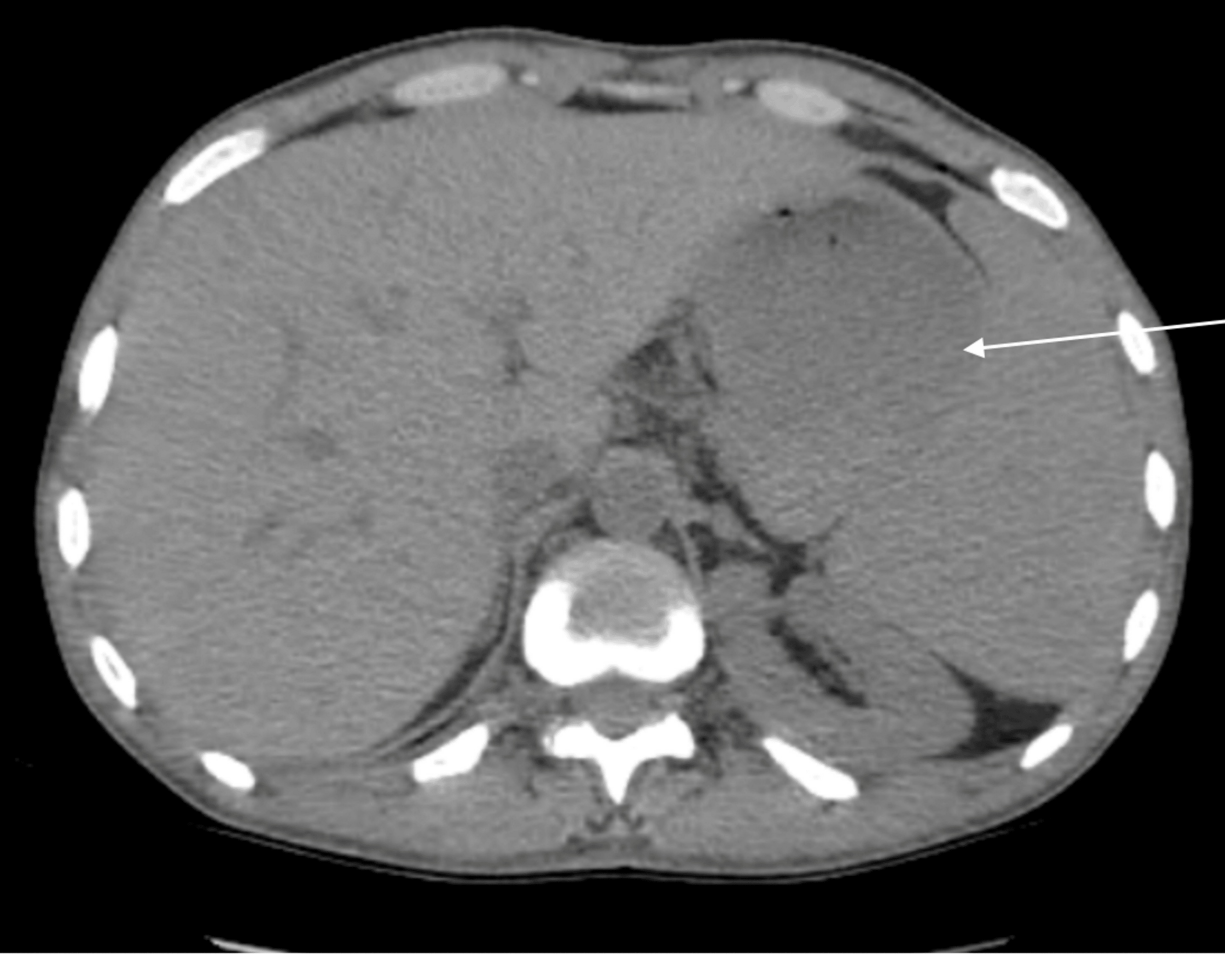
Axial CT urogram image demonstrating a large left mid-renal mass
